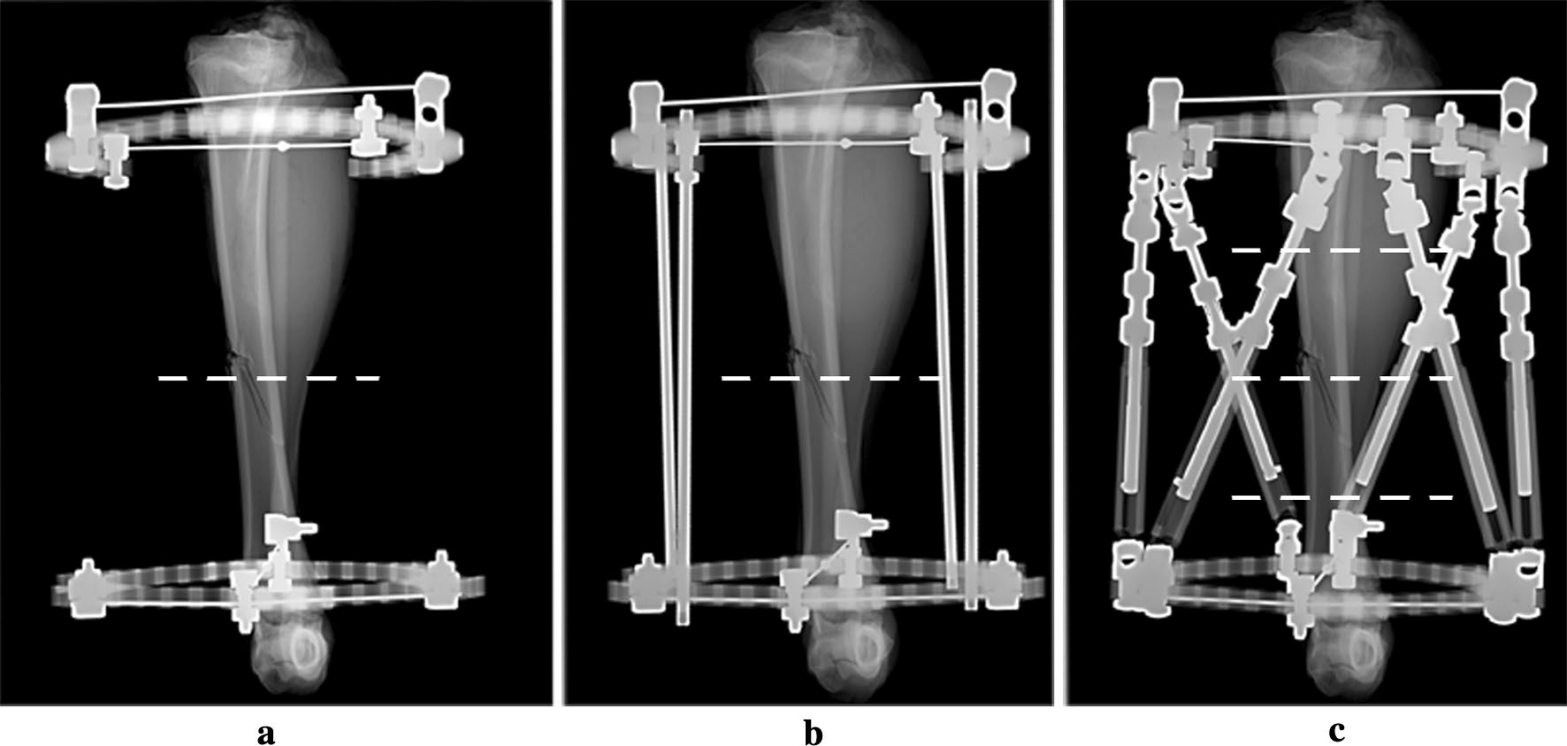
X-rays (anterior–posterior views) showing various frame constructs. a Initial positioning of the rings. b Ilizarov frame (4 rods). c Taylor Spatial Frame. Dotted lines show the position of the slices used for the study
